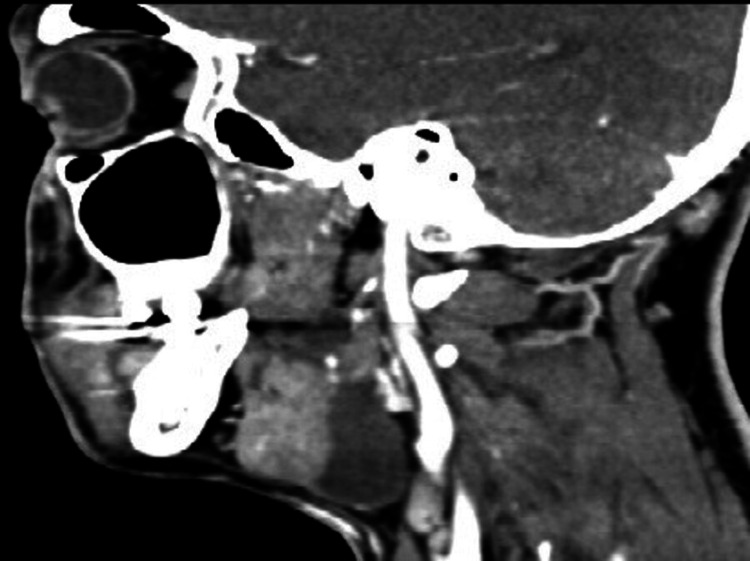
CT sagittal view of lesion showing posterior inferior extension abutting the right carotid artery and close relation to right submandibular gland.
